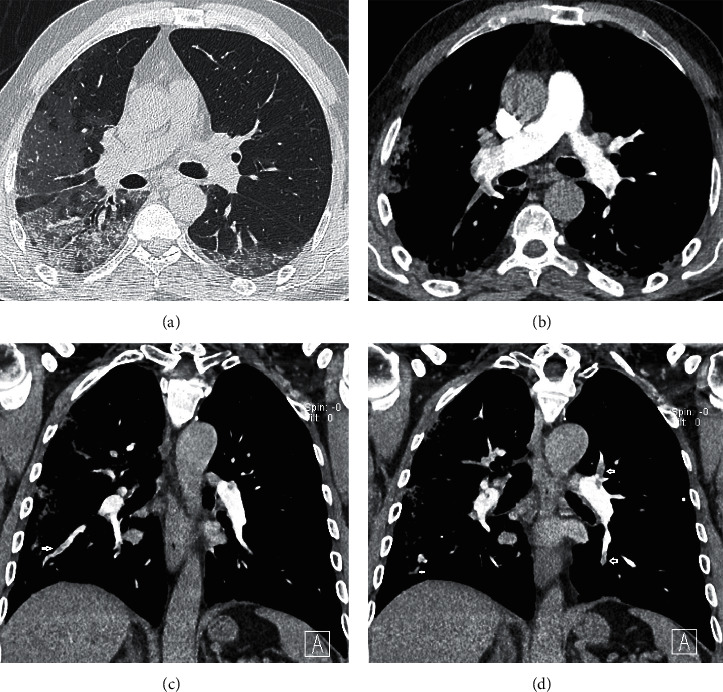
Images in a 64-year-old man with COVID-19 pneumonia complaining of body ache, fever, and cough. (a) Axial unenhanced chest CT scan (lung window) obtained on day 9 after the onset of symptoms shows bilateral areas of peripheral ground-glass opacities more at the right side with interlobular septal thickening (crazy paving sign), vascular sign, and air bronchogram sign being seen. (b) Axial CT pulmonary angiography scan. (c, d) Coronal reformatted images (mediastinum window) on day 24 after the onset of symptoms demonstrate multiple bilateral filling defects (white arrows) involving lobar, segmental, and subsegmental branches of the pulmonary artery.
